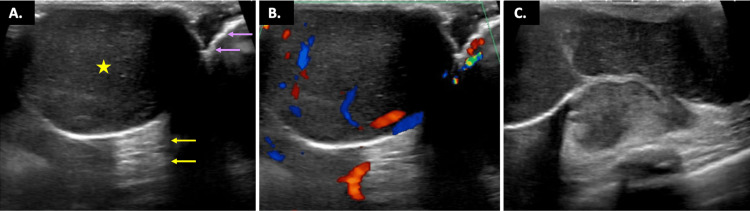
Sonographic Examination Findings in the Left Breast Image 3A, taken at 7:00, 5 cm from the nipple in the left breast, demonstrates a large, oval, circumscribed, parallel-oriented, hypoechoic mass (yellow star) with acoustic enhancement (yellow arrows) noted behind it. The dermal layer (lavender arrows) can also be seen cradling the mass, as it appears to invade from the dermis into the deeper subcutaneous tissues. Image 3B shows increased vascularity within the peripheral regions of the mass, in the same location in the left breast. Image 3C reveals a different view at 5:00, 5 cm from the nipple, depicting the lobulated appearance of this patient’s advanced DFSP left breast lesion.
